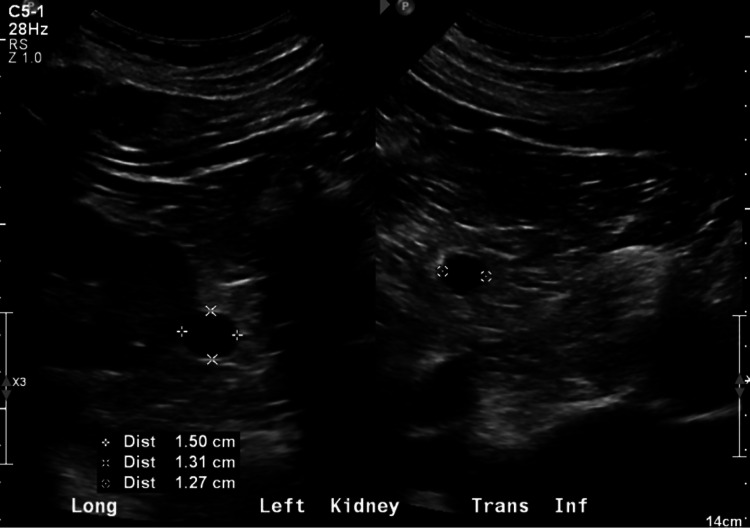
The patient was noted to have multiple renal cysts bilaterally. The largest cyst noted on a left kidney ultrasound measured 1.5 cm x 1.31 cm x 1.27 cm.
